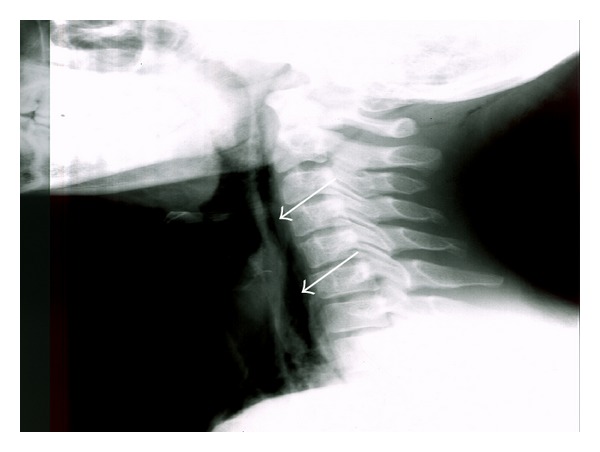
Lateral radiograph of the neck showing extensive subcutaneous emphysema (arrows).
